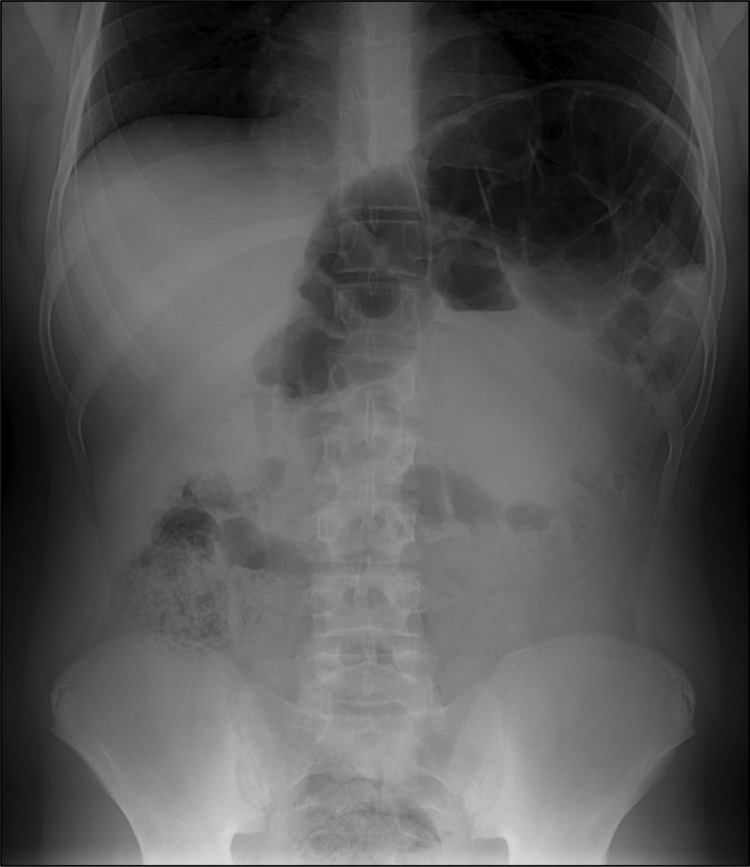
Abdominal radiograph of a 13-year-old boy with congenital Chagas disease, Japan, showing megacolon and marked dilatation at the splenic flexure.
